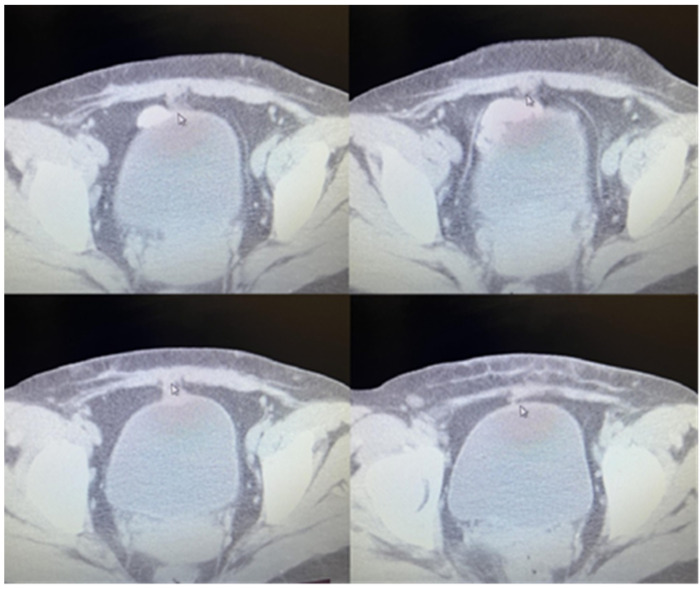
Computed tomography in transverse sections.
